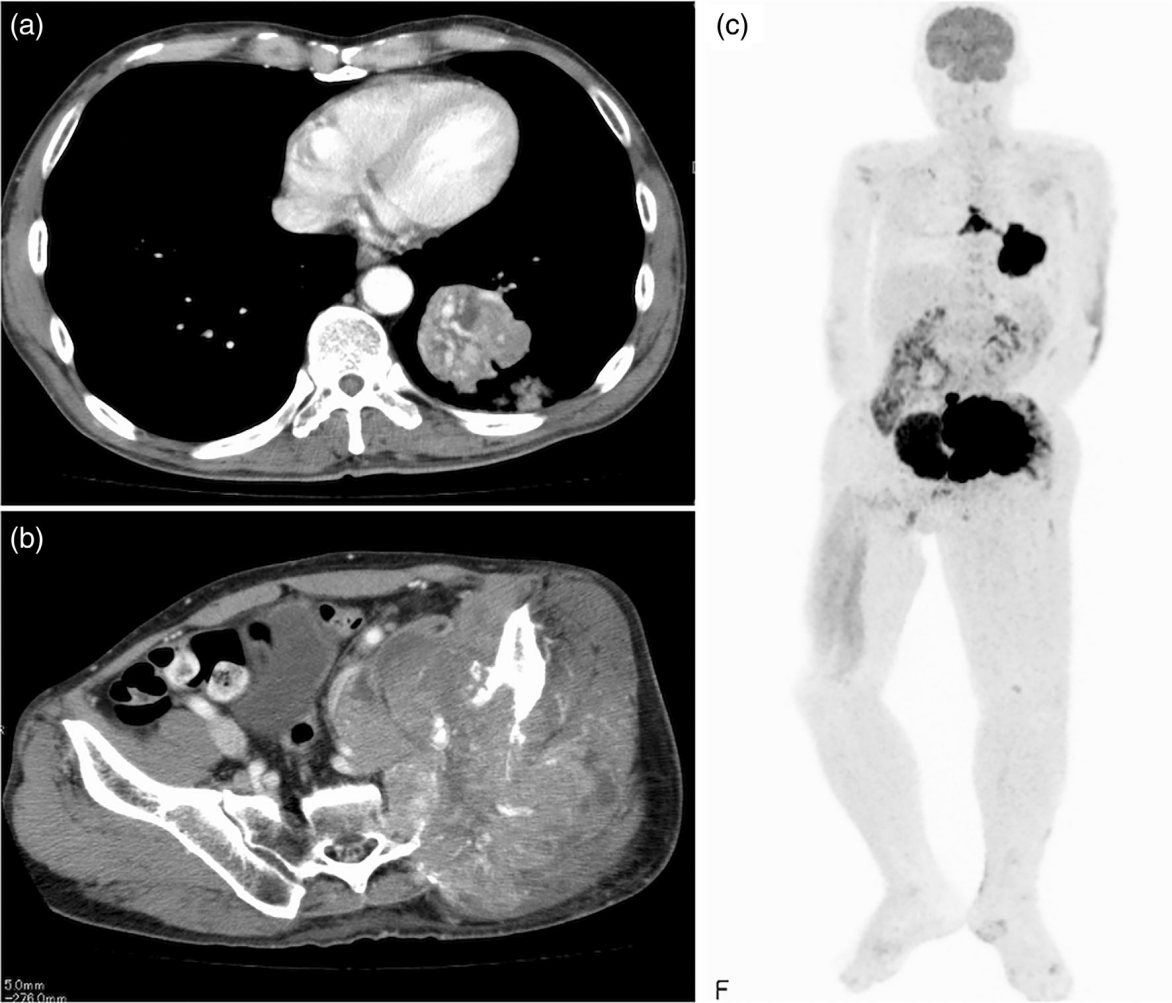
Contrast CT and PET‐CT. (a) A mass lesion 51 mm in length with an angiogram sign was observed in the lower lobe of the left lung. Peripherally to the same mass, a 47 mm long mass and a significantly enlarged ipsilateral hilar lymph node were observed. (b) A 146 mm long mass was found in the left iliac bone, extending into the pelvic cavity and muscle layer. There was no invasion into the skin or subcutaneous tissue. (c) PET showed abnormal FDG accumulation consistent with a left lung mass, mediastinal and left hilar lymph nodes, and a left iliac mass. No abnormal FDG accumulation was found in other areas. CT, computed tomography; FDG, fluorodeoxyglucose; PET‐CT, positron emission tomography.
